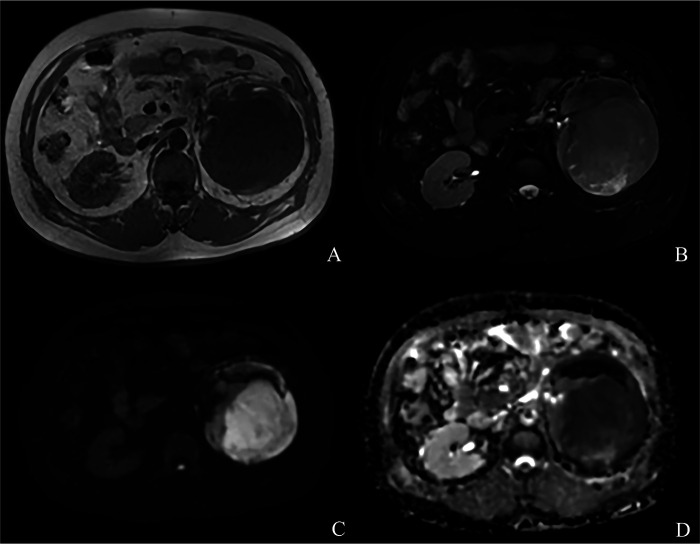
MRI of both kidneys: (A) slightly low signal on T1WI (B) slightly high signal on T2WI (C) high signal on DWI (D) ADC was decreased.
